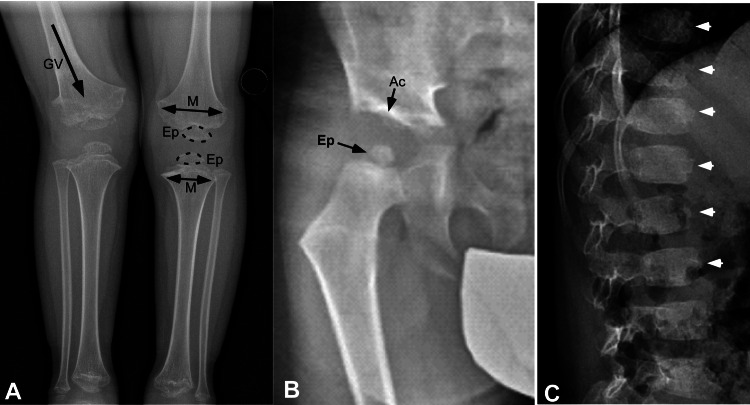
Radiographic findings are consistent with pseudoachondroplasia. (A) Knee image shows small irregular epiphysis (Ep), widened metaphysis (M), and genu valgum (GV). (B) Hips have underdeveloped acetabulum (Ac) and capital femoral epiphyses. (C) Vertebrae show platyspondyly with anterior beaking (marked by white arrowheads).
